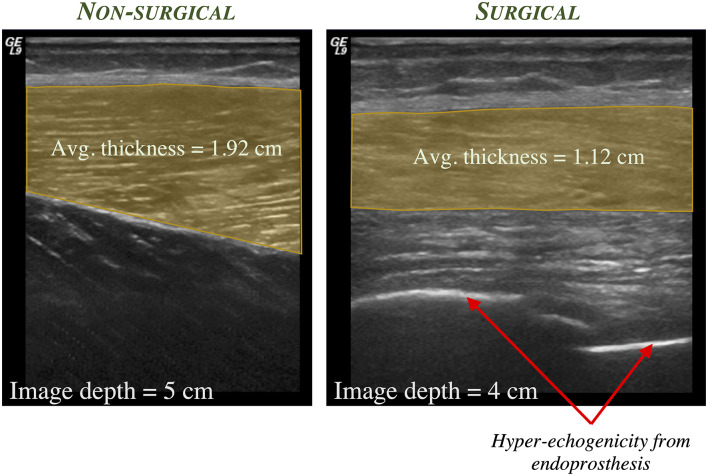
Representative static 2-D ultrasound images of the anterolateral thigh in a 17-years old male who was diagnosed at age 11 with osteosarcoma of the distal femur and underwent subsequent limb salvage surgery with Stanmore endoprosthesis placement and lengthening. The thickness of the vastus lateralis muscle (shaded region) is greater in the non-surgical limb (left) compared to the surgical limb (right).
